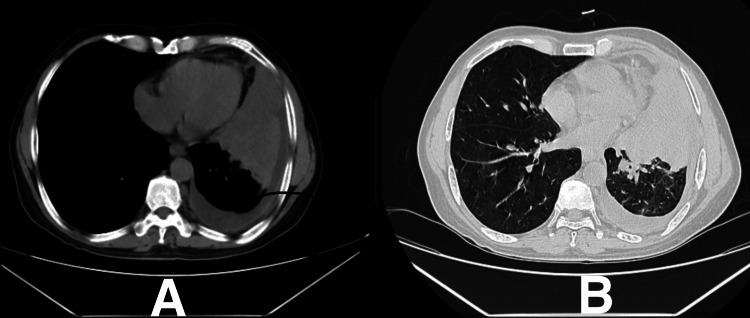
High-resolution computed tomography (HRCT) of the thorax showing volume loss due to a collapsed segment of the left lung, ipsilateral mediastinal shift due to central mass lesion, and centrilobular emphysematous changes over bilateral upper lobes. A: HRCT mediastinal window of the thorax. B: HRCT lung window of the thorax.
